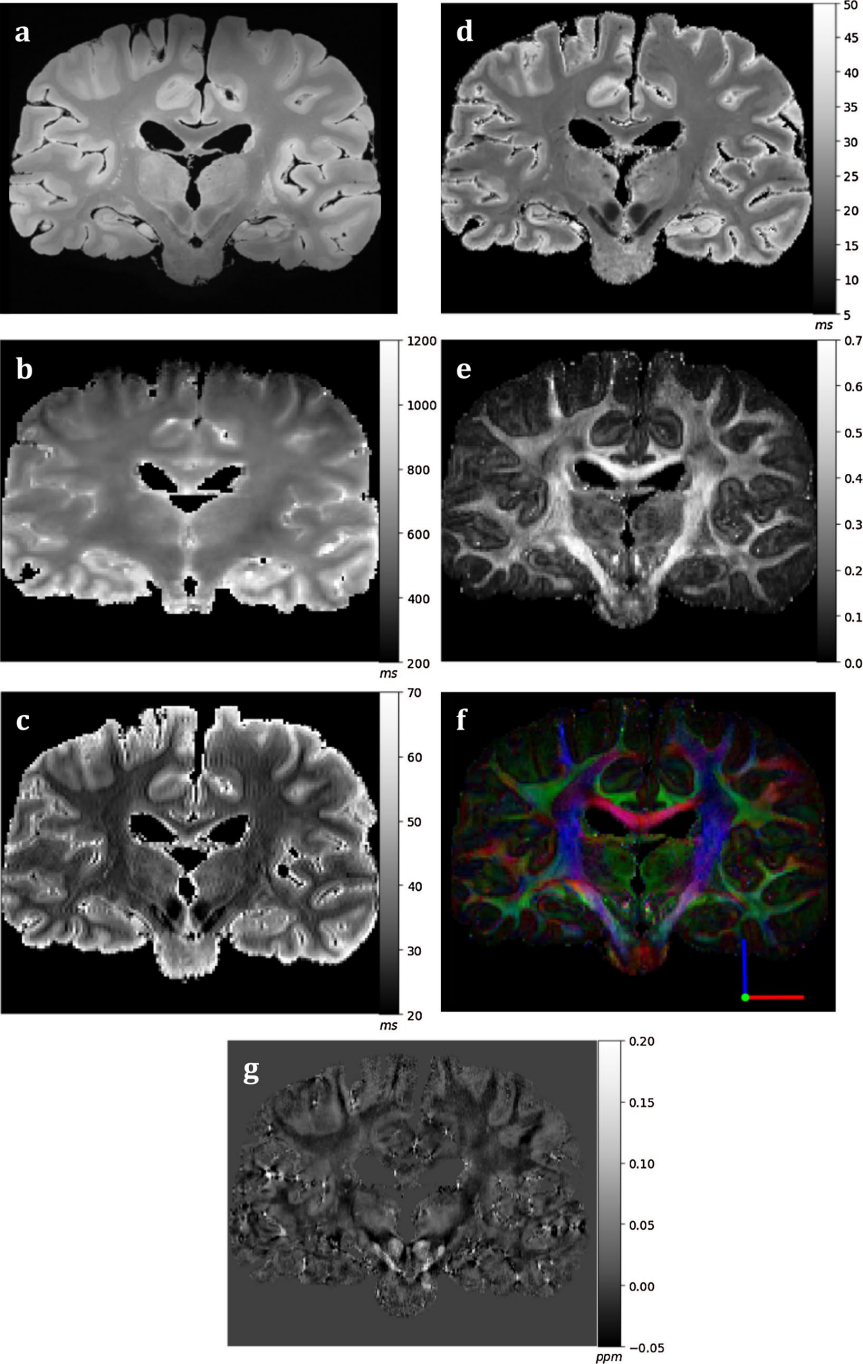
Processed MRI datasets from an individual brain. The coronal MR images display a structural map (a), T1-map (b), T2-map (c), T2*-map (d), FA map (e), principal diffusion direction weighted by the FA (f) and susceptibility map (g). The calibration bars for the T1-map (b), T2-map (c) and T2*-map (d) indicate the T1, T2 and T2* values (in ms), respectively. The calibration bar in the FA map (e) indicates the fractional anisotropy. The key at bottom right of f indicates colour orientation of the principal diffusion direction. The calibration bar in the susceptibility map (g) indicates the susceptibility in parts-per-million (ppm)
